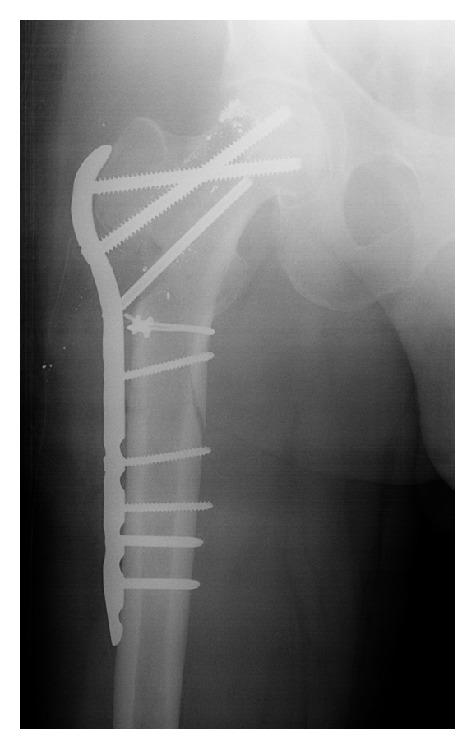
Right hip radiograph after open reduction and internal fixation.
